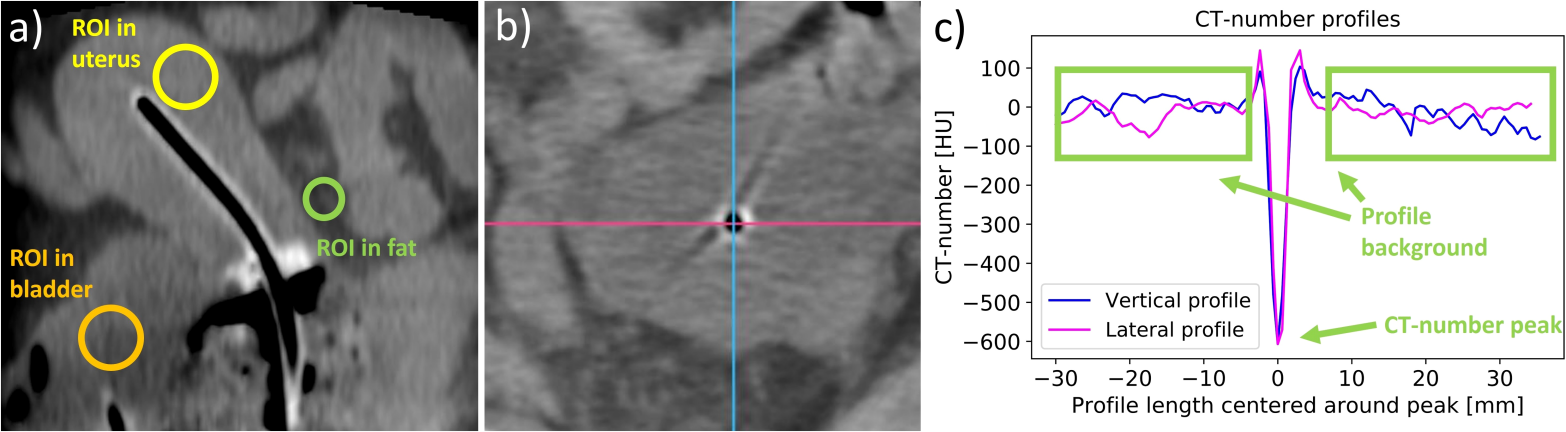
The placement of regions of interest (ROIs) in the uterus, the bladder, and the surrounding fat tissue (a), which were used to determine the contrast–noise ratio. Furthermore, lateral and vertical CT number profiles were measured in the regions of the applicator tips (shown in panel (b) as an example of an intrauterine probe) to evaluate the visibility of the applicators. The two directions are exemplified by a lateral pink line and a vertical blue line. The CT number of the peak at the applicator position and the mean value of the CT numbers of the profile background were then calculated from the created profiles (c), and their difference was determined.
